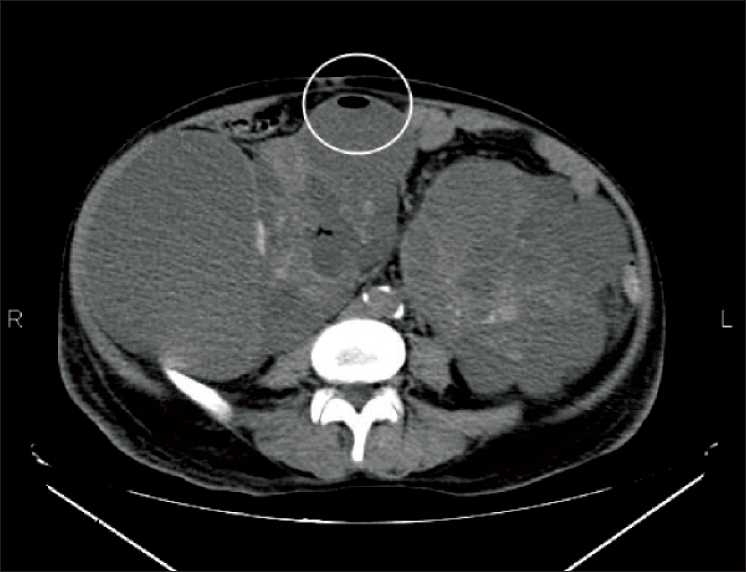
CT abdomen of the patient showing bilateral enlarged kidneys with multiple cysts. One of the cysts in the right kidney shows an air bubble (see circle)
